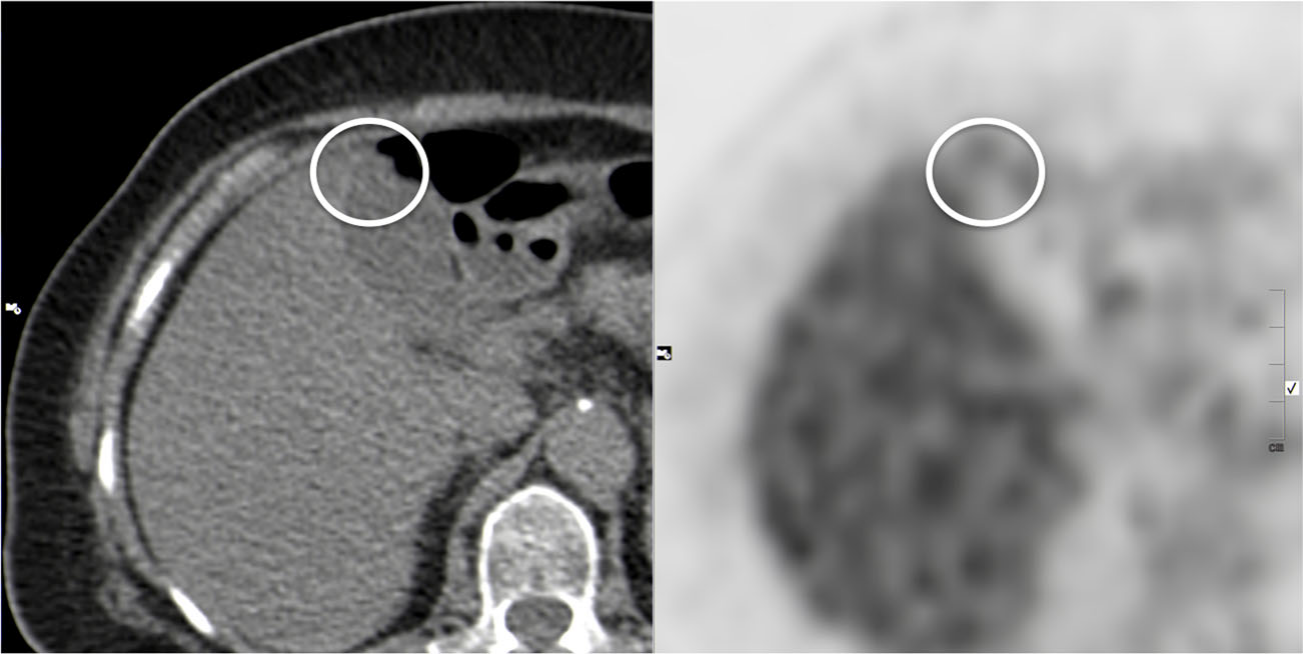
Gallbladder adenomyomatosis: typical PET-CT findings. Gallbladder adenomyomatosis (*circle*) usually shows an F-18FDG uptake equal or lower than the adjacent liver
